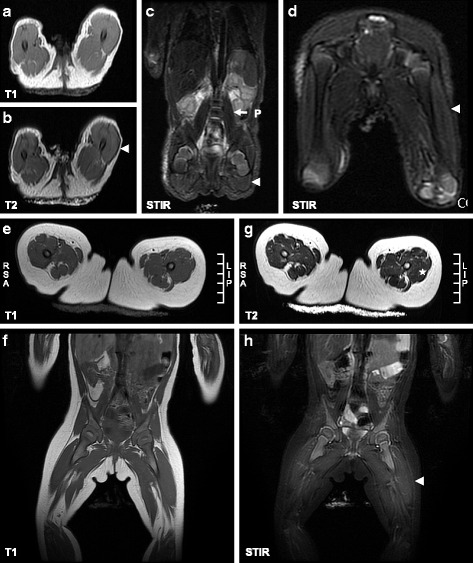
Earliest muscle MRI changes in newborn patients with IOPD. a-d At 13 days of age, no significant signal changes in the thigh were revealed on T1WIs (a) obtained from NBS14, with equivalent findings on T2WIs (b). However, significant signal changes were observed on STIR images (c, d), which revealed hyperintensity, particularly in the vastus lateralis (arrowhead). e-h At 7 months of age, hyperintensity was noted in the anterior region of the thigh muscles on T2WIs (G, asterisk) and STIR images (h, arrowhead) but not on T1WIs (e, f)
